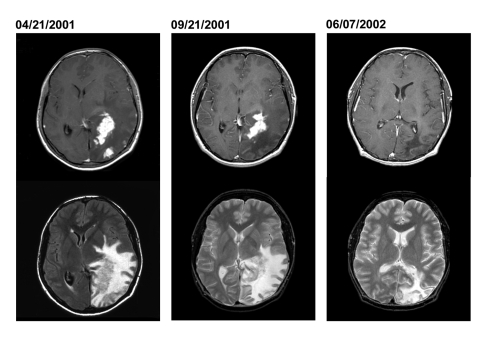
Axial MRI (magnetic resonance imaging) from patient 2 obtained when first seen in Peru (April 21, 2001), before surgical biopsy in the United States (September 21, 2001), and 7 months after start of treatment (June 7, 2002). The top images are postgadolinium-enhanced, T1-weighted images, which demonstrate resolution of one of the irregular areas of enhancement over time. The bottom images are T2-weighted images (image from April 21 is from a fluid attenuated inversion recovery [FLAIR] sequence), which demonstrate the extensive brain edema associated with these lesions. The right bottom image shows resolution of the brain edema but persistent brain atrophy.
